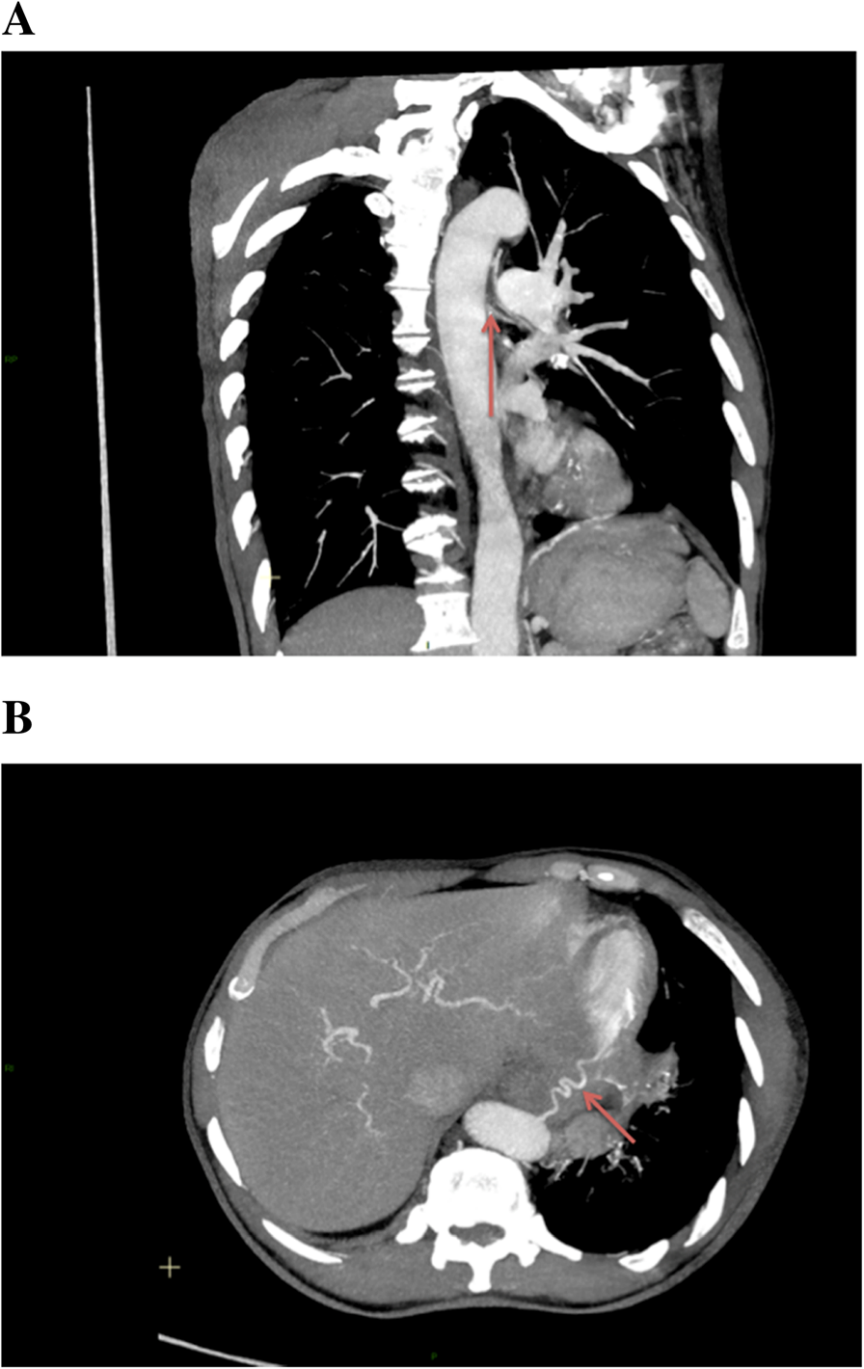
a Coronal CT section showing enlarged left bronchial artery (red arrow). b Axial CT section showing enlarged left phrenic artery (arrow)
